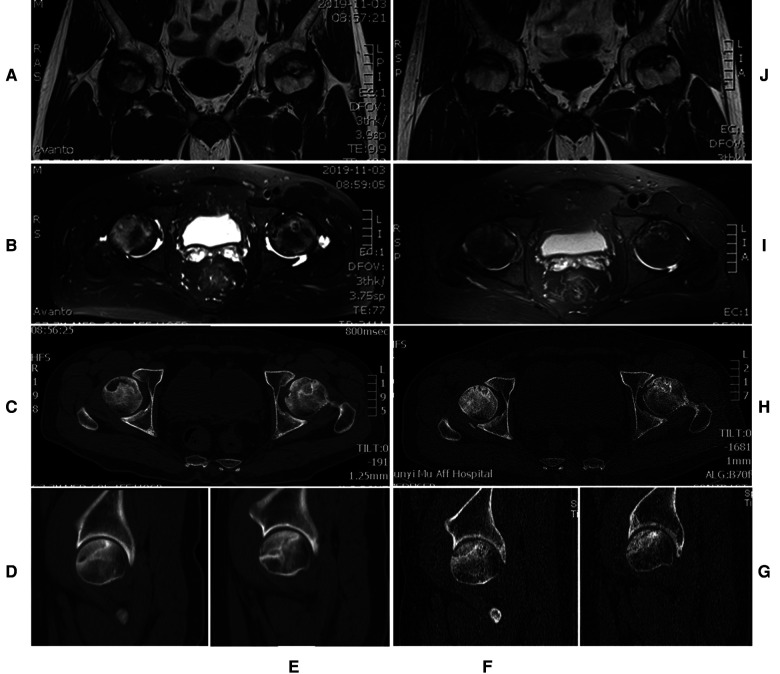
The preoperative axial MRI shows an area of a low-intensity signal on a T1-weighted image (A) and a high-intensity signal on a T2-weighted image (B). The preoperative axial (C) and sagittal (D, right side) (E, left side) CT scans show cystic and sclerotic changes at the anterosuperior area of the bilateral femoral heads. Postoperative MRI on T1-weighted (J) and T2-weighted (I) images show a decrease of cysts and oedema in the bilateral femoral heads. The postoperative axial (H) and sagittal (F, right side) (G, left side) CT scans show that the necrotic site is partly replaced by a new bone.
